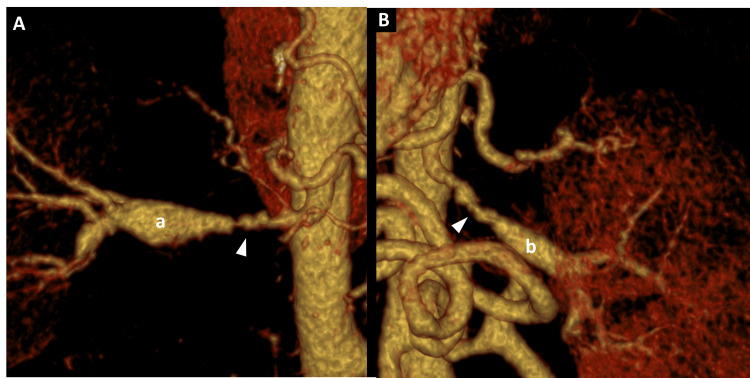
Three-dimensional reconstruction of CT angiography of the renal arteries. 3D coronal view of the right renal artery (A) and left renal artery (B) demonstrating fibromuscular dysplasia with the characteristic “string-of-beads” appearance (arrowheads). a: right renal artery; b: left renal artery.
